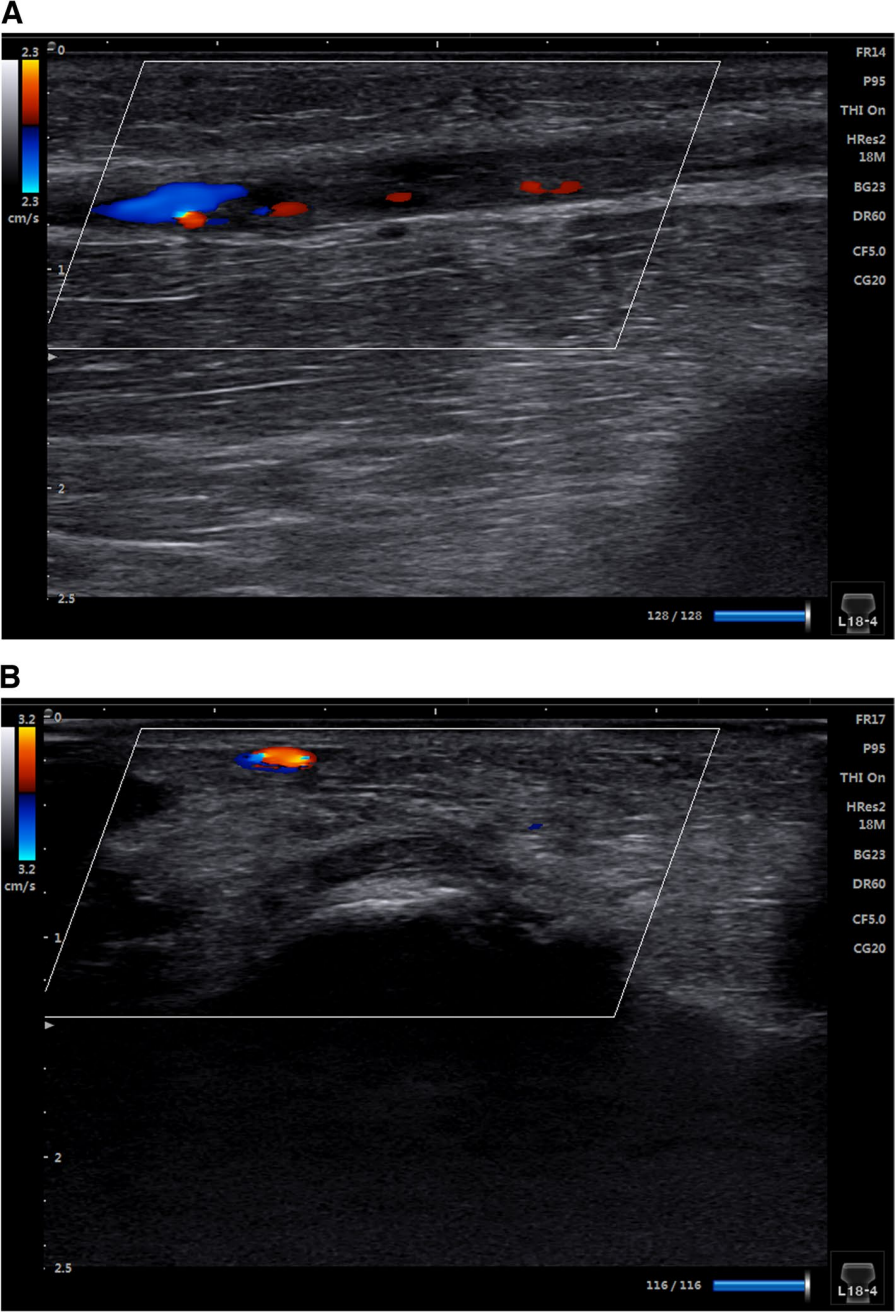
Ultrasonography of RAO and dRAO. a Ultrasonography of RAO. b Ultrasonography of dRAO
